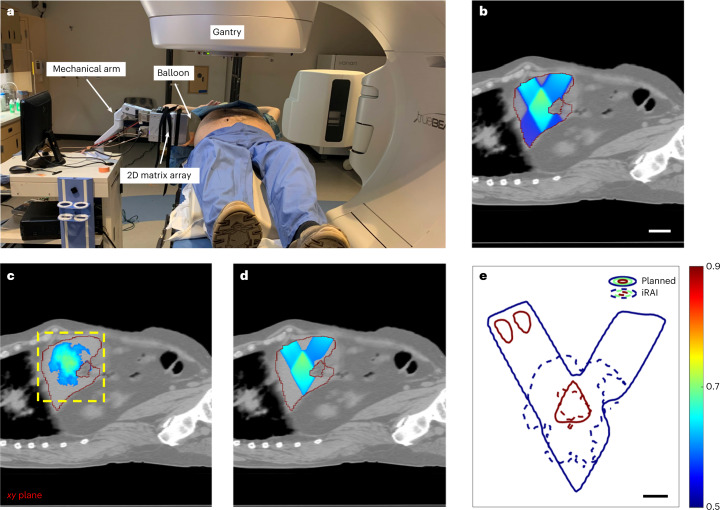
In vivo iRAI imaging versus the treatment plan on a patient. a, A photograph of the iRAI imaging on a patient taken during RT. b, The dose distribution of only the two static sagittal beams of the treatment plan with a liver mask fused onto the CT scan anatomy structure. Scale bar, 5 cm. c, The iRAI measurement of dose with a liver mask fused onto the CT anatomy structure with the same position as b. The yellow dashed box indicates the field of view of the 2D matrix array. d, Dose distribution (>50%) of the treatment plan with a liver mask fused on the CT anatomy structure. e, The 50 and 90% isodose lines in the iRAI measurement and the treatment plan. Scale bar, 2 cm. The red line in b–d indicates the boundary of the liver.
